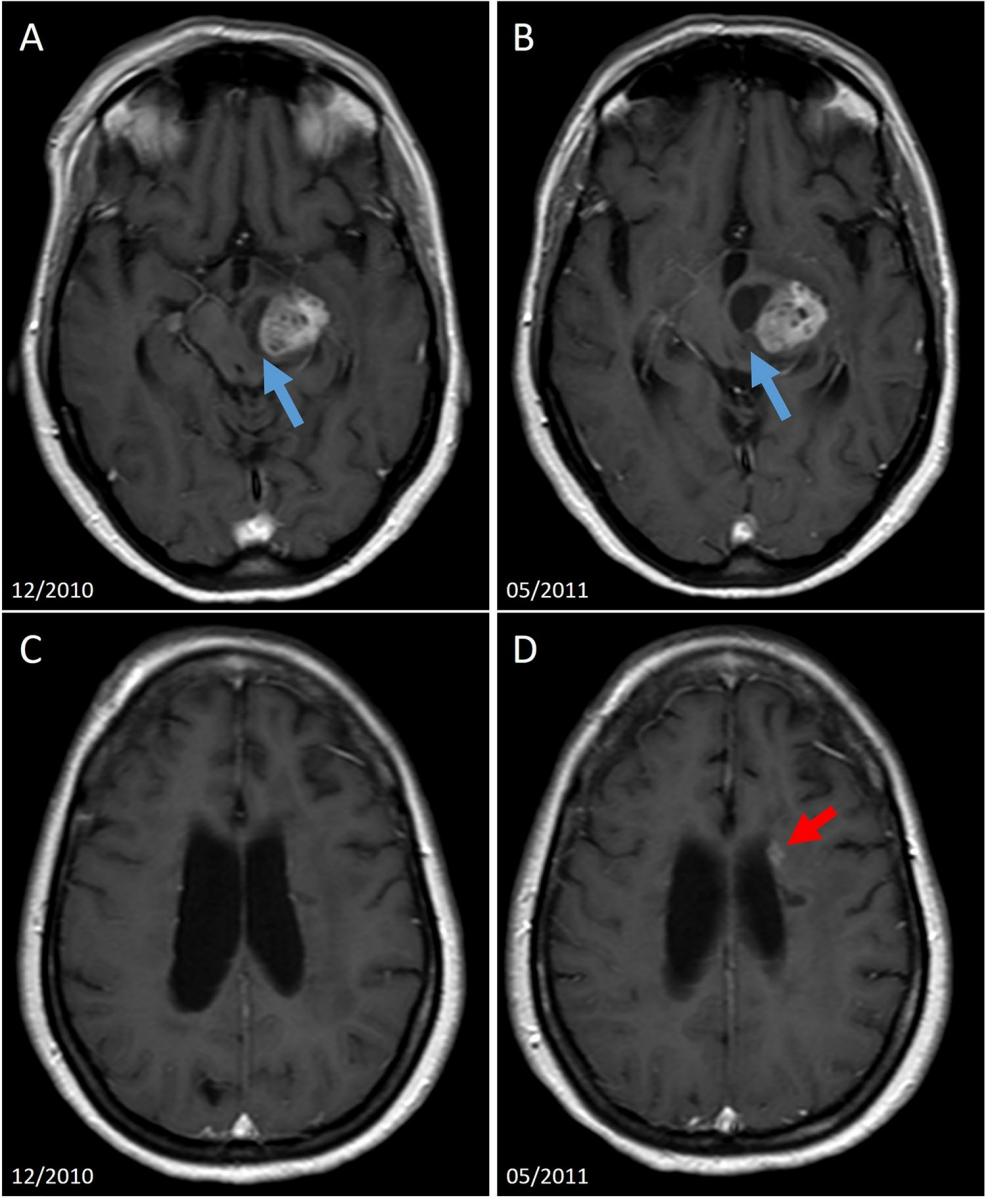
Pseudoprogression in concomitant MS and CNS tumor. A This 46-year-old female patient was diagnosed with a pilocytic astrocytoma affecting the left thalamus and the left cerebral peduncle. Relapsing–remitting MS had been diagnosed 11 years before that. She was treated with radio- and chemotherapy for the astrocytoma. During one of the 6-month follow-ups, she reported new dysarthria and increasingly impaired ambulation. Brain MRI revealed that the cystic portion of the tumor has grown (A–B, blue arrows) affecting more strongly the left cerebral peduncle. Additionally, a new periventricular gadolinium-enhancing MS lesion has appeared, which was not seen on the previous brain MRI (C–D, red arrow). Because of increasingly pronounced symptoms and further growth of the cystic portion, the cystic portion was punctured. No corticosteroid therapy was initiated for the new MS lesion. The lesion was not present on the further follow-ups anymore (not shown here)
